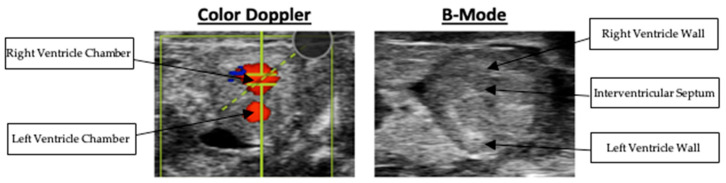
Color Doppler and B-Mode scans of right ventricular outflow tract velocity.
